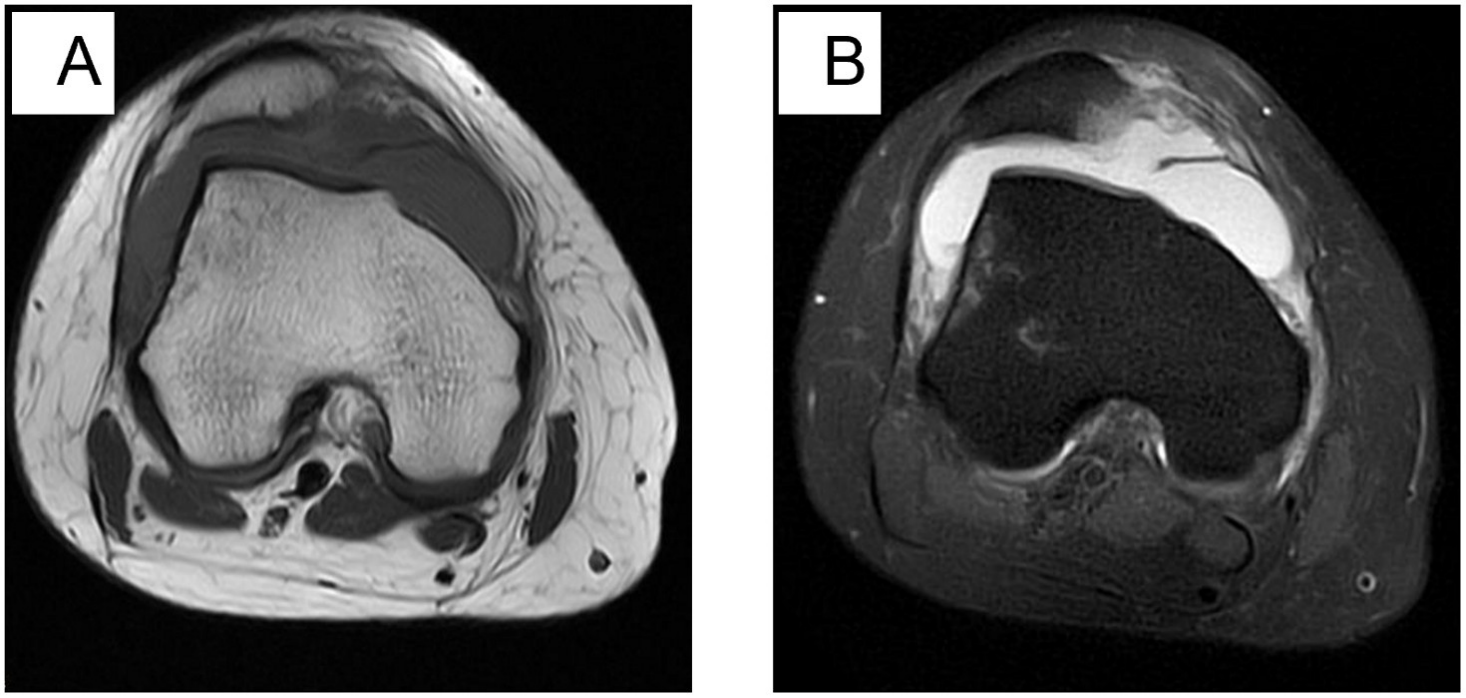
A: Axial T1-weighted MR image; B: Axial fat-suppressed proton density-weighted MR image. Female, 26 years old, tangential fracture. Patellar subluxation with localized osteochondral defects in the medial portion of the patellar, patchy osteoedema of the lateral femoral condyle on axial fat-suppressed proton density-weighted MR image, and grade II signal in the medial patellar retinaculum. Simple effusion in the joint capsule.
